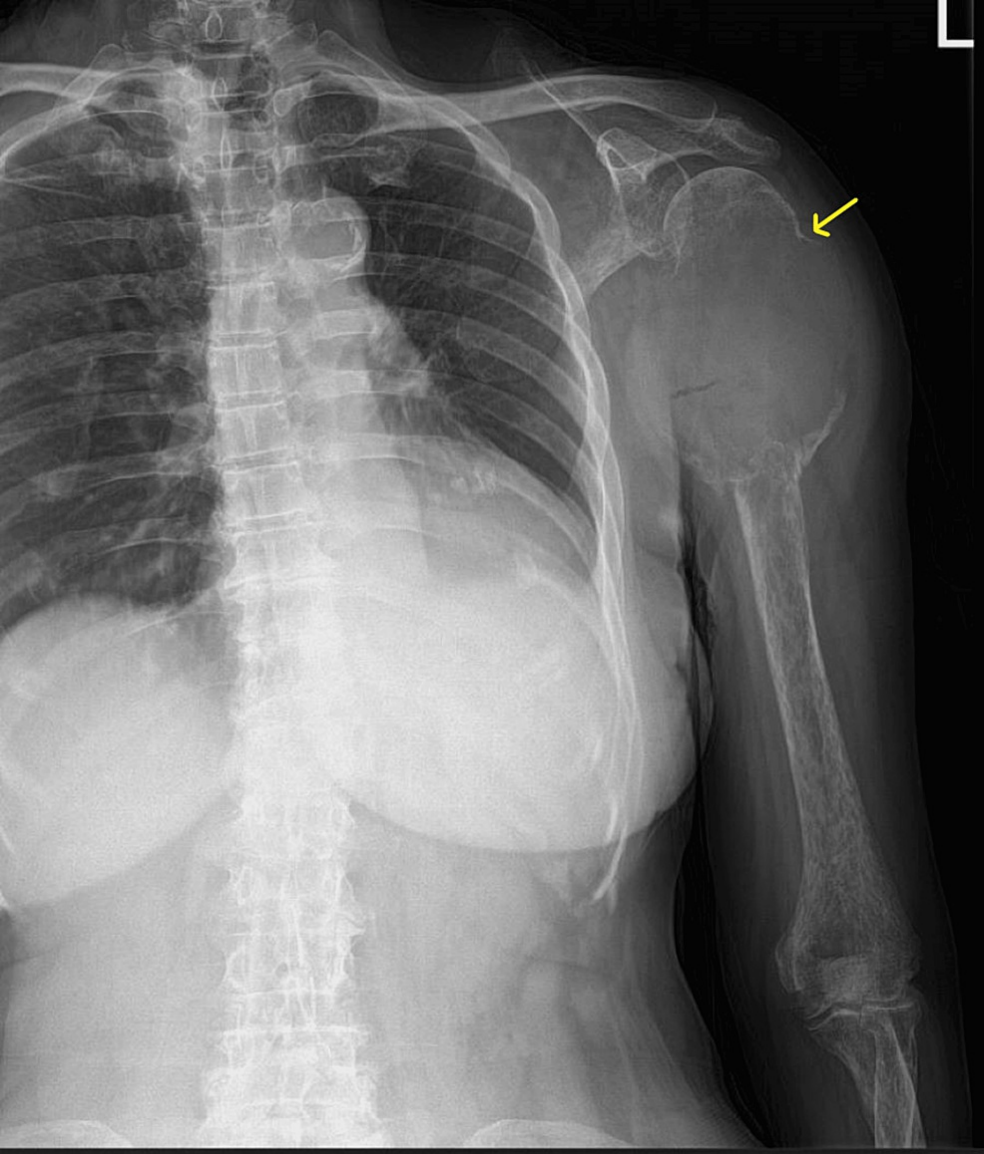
A 73-year-old female patient with oncocytic cancer presented with left shoulder-humerus metastasis, as observed on a direct radiograph.
